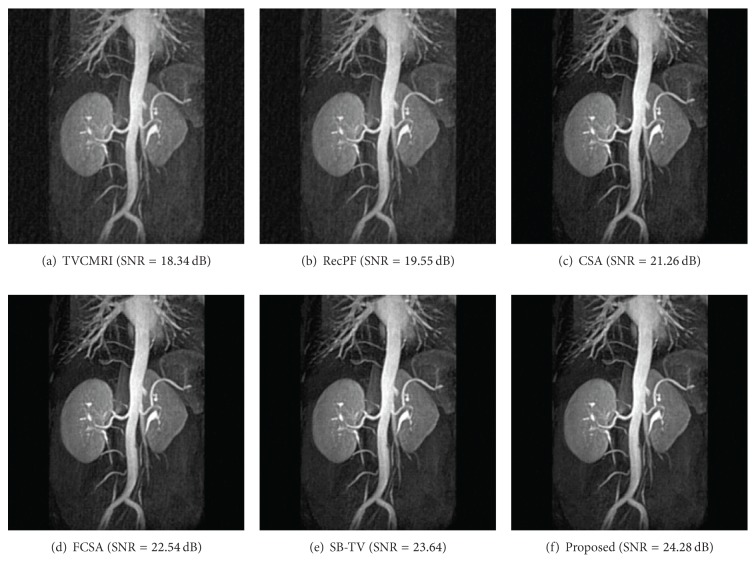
Artery MR image reconstruction from 20% samples (Rician noise case).
